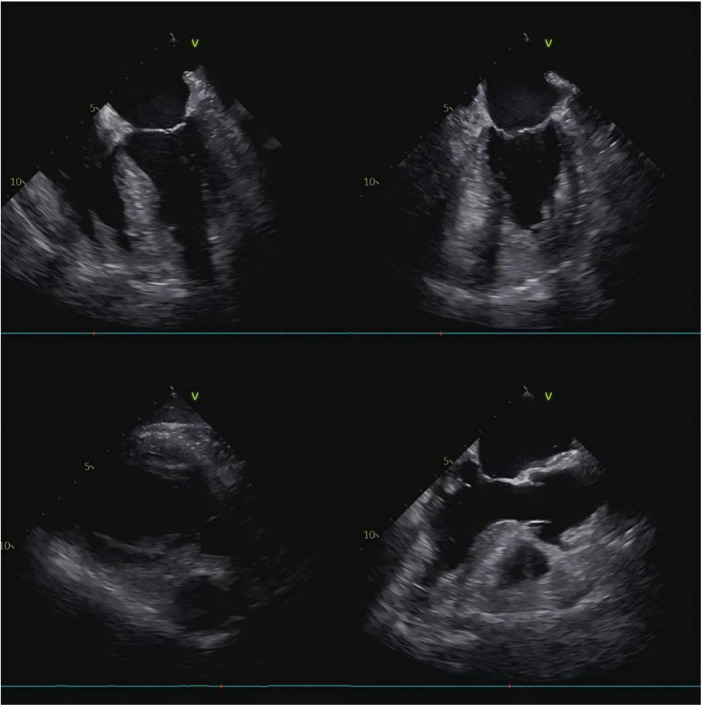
Postoperative transesophageal echocardiography showing no residual mass in left ventricular cavity from multiple angles.
